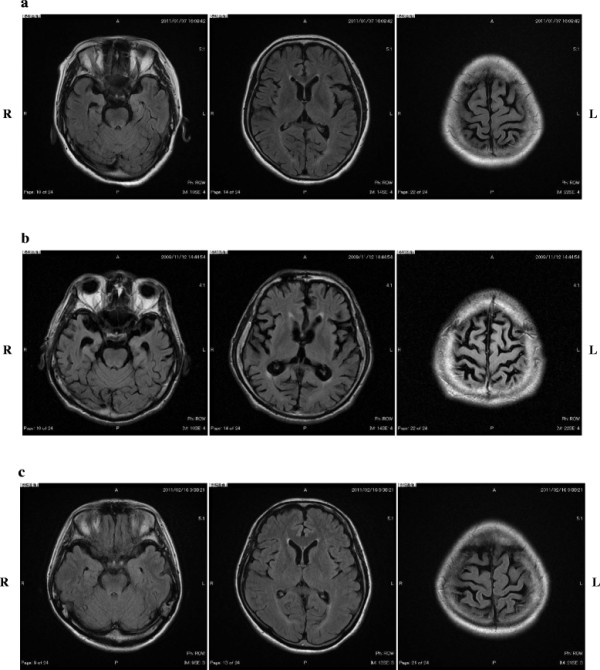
Brain MRI (FLAIR). a) III-9 at the age of 55 years, b) the proband (III-10) at the age of 53 years, c) III-11 at the age of 52 years. All siblings had generalized cerebral atrophy but this was mild relative to their clinical features.
